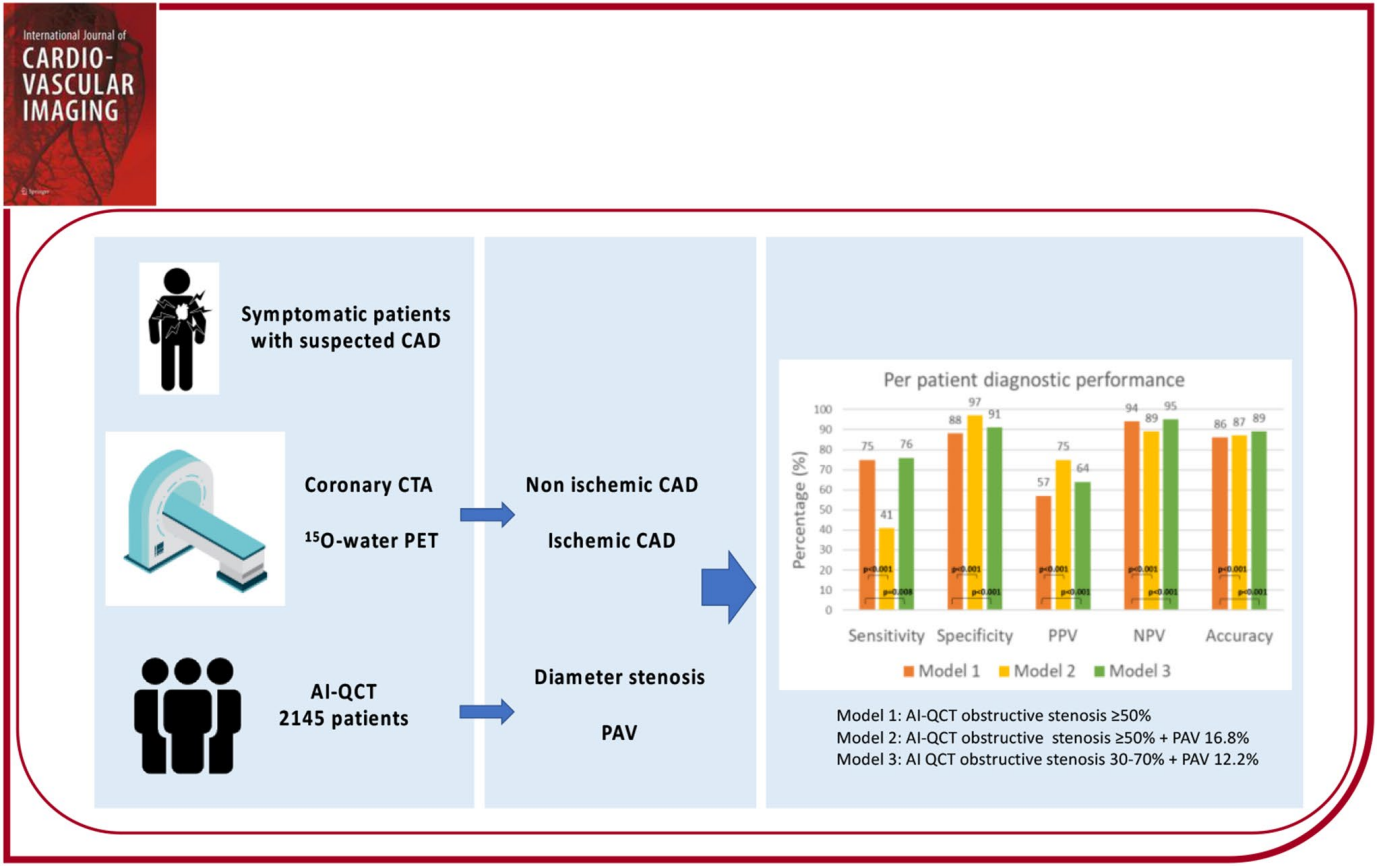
Symptomatic patients with suspected CAD underwent coronary CTA and selective ^15^O-water PET myocardial perfusion imaging. Adding AI-QCT plaque burden improved the detection of ischemic CAD in comparison with diameter stenosis only. CAD = coronary artery disease, CTA = computed tomography angiography, PET = positron emission computed tomography, AI-QCT = artificial intelligence-guided quantitative computed tomography, PAV = percent atheroma volume
